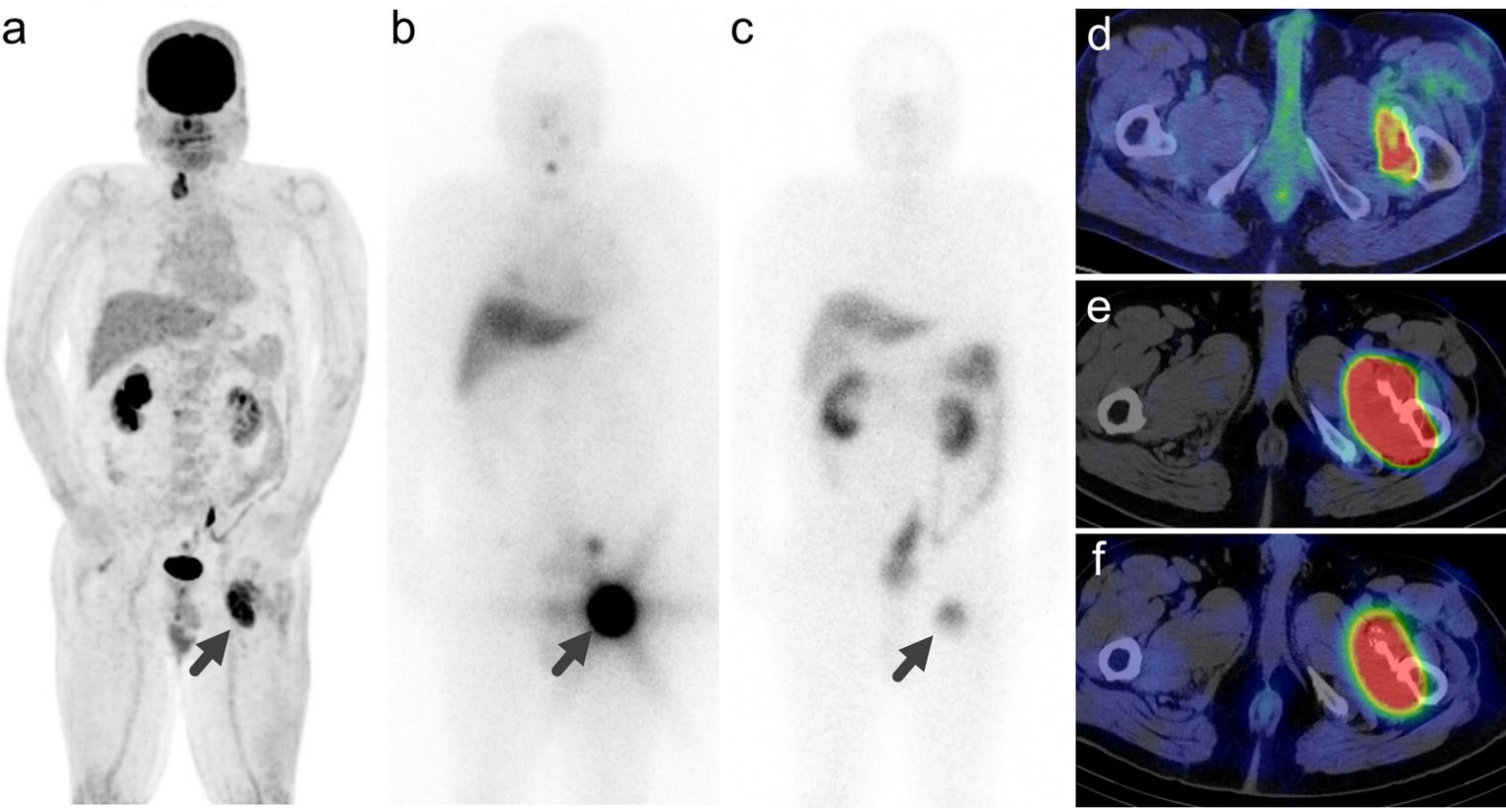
Representative case 1. A case of a man in his 60s with follicular thyroid carcinoma and metastasis to the left femur. (a) Maximum intensity projection of ^18^F-FDG PET showing increased uptake corresponding to the left femoral metastasis (Score 5/5, SUVmax = 9.1). (b) Post-treatment ^131^I scintigraphy performed at 10 days after ^131^I administration demonstrated significant iodine uptake (Score 3/3, SUVmax = 33.7). (c)^111^In-pentetreotide scintigraphy showing intense uptake, stronger than that in the liver (Score 3/4, SUVmax = 5.4). (d) Fused image of ^18^F-FDG PET and CT. (e) Fused image of ^131^I SPECT and CT. (f) Fused image of ^111^In-pentetreotide SPECT and CT
